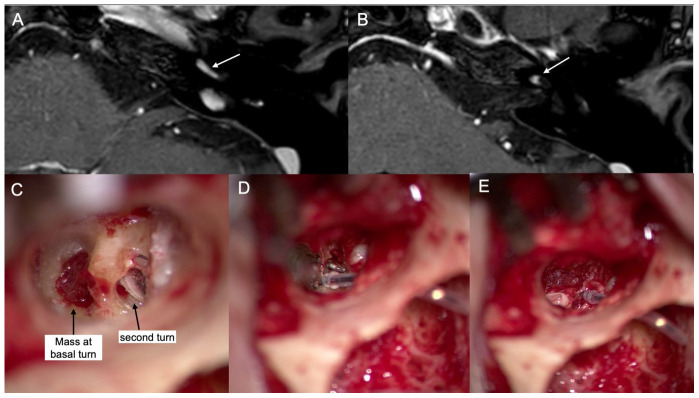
Case #3 (A–E). Preoperative T1-weighted MR with gadolinium enhancement shows the enhancing mass in the basal turn (A) and second turn (B) of the cochlea (white arrows). Operative findings (C–E): mass at the basal turn and second turn of the cochlea (black arrows) (C), electrode in the basal and second turns (D), and repair of the cochlea with fascia and cartilage (E).
